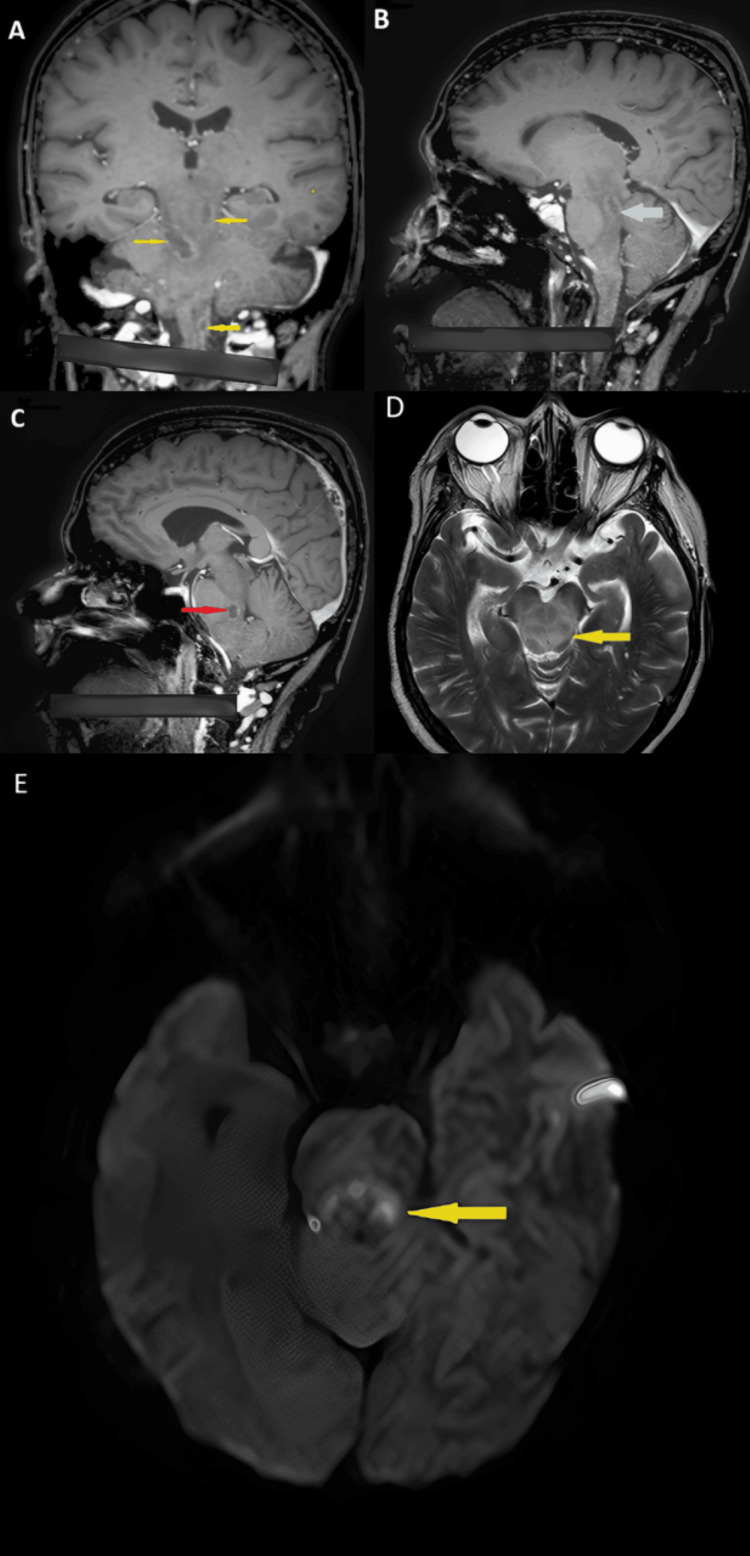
(A) Coronal T1 MR image with contrast of the patient’s brain. Yellow arrows indicate multiple lesions in the midbrain, pons, and upper cervical spinal cord. These lesions demonstrate peripheral ring enhancement with central non-enhancement, typical of tunneling abscesses. Right side is indicated. (B) Sagittal T1 MR image with contrast. Light blue arrow shows a lesion in the upper pontine tegmentum with peripheral ring enhancement and central non-enhancement. (C) Sagittal T1 MR image with contrast. Red arrow shows a lesion in the lower pontine tegmentum with central low intensity and peripheral ring enhancement. (D) Axial T2 MR image. Green arrow indicates midbrain hyperintensity consistent with edema. (E) Axial diffusion-weighted MR image. Yellow arrow demonstrates diffusion restriction within small ring-enhancing lesions, consistent with microabscesses.
